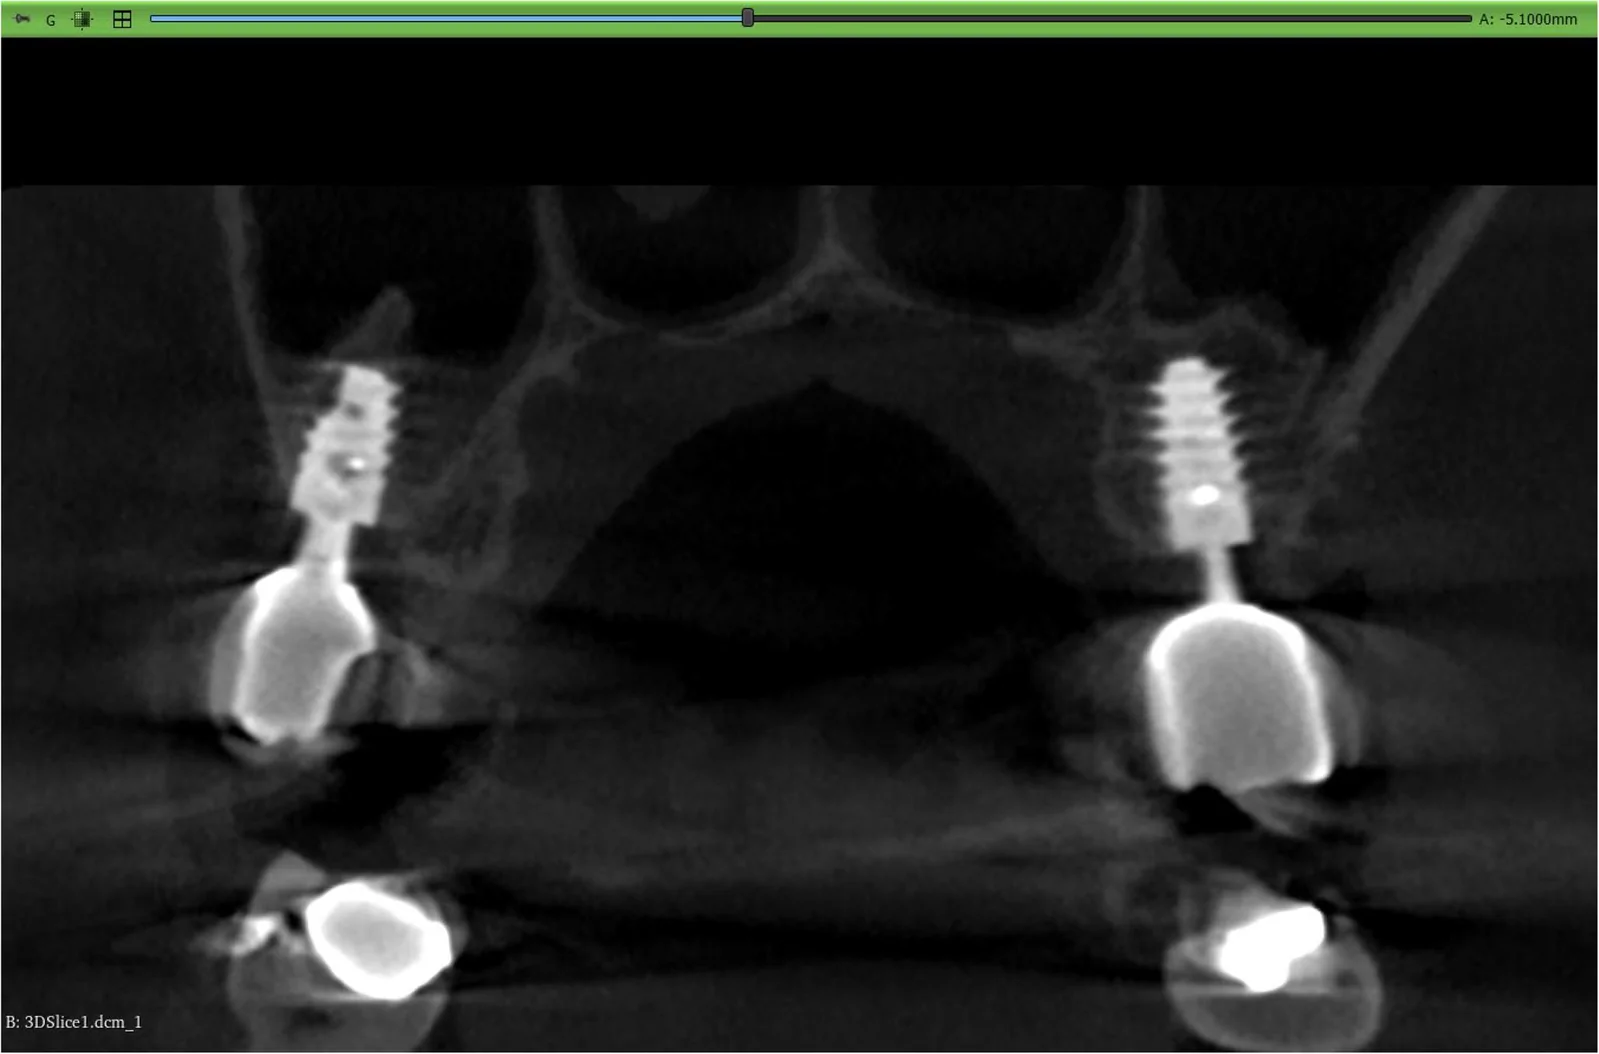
An example of 1-year CBCT evaluation
